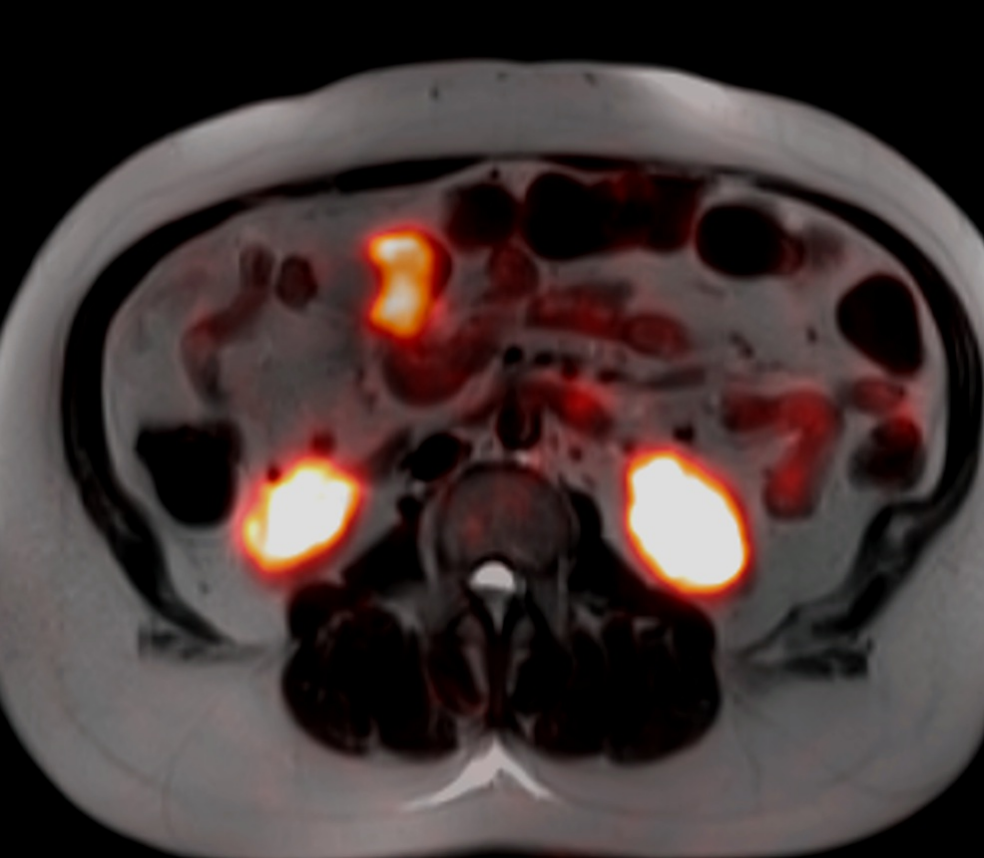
Fused axial image from gallium dotatate PET-MRI demonstrating focal radiotracer uptake associated with the loop of small bowel represent primary small bowel carcinoid PET, positron emission tomography; MRI, magnetic resonance imaging.
